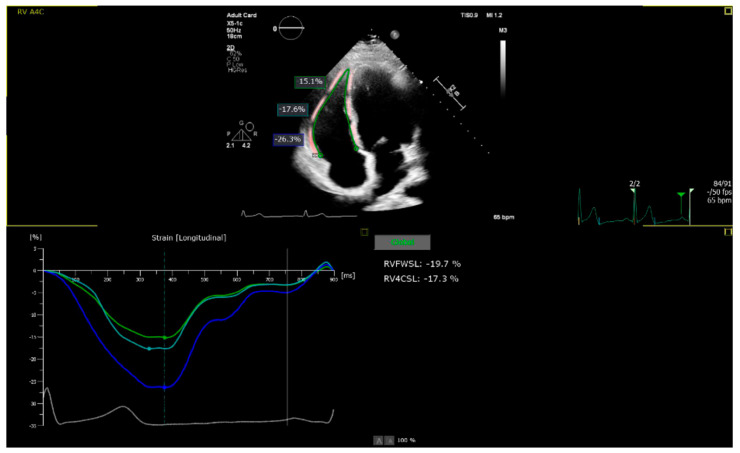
Apical right ventricular—focused four-chamber view obtained by two-dimensional echocardiographic acquisitions and automatic analysis of the right ventricular strain using two-dimensional speckle transthoracic echocardiography (authors).
